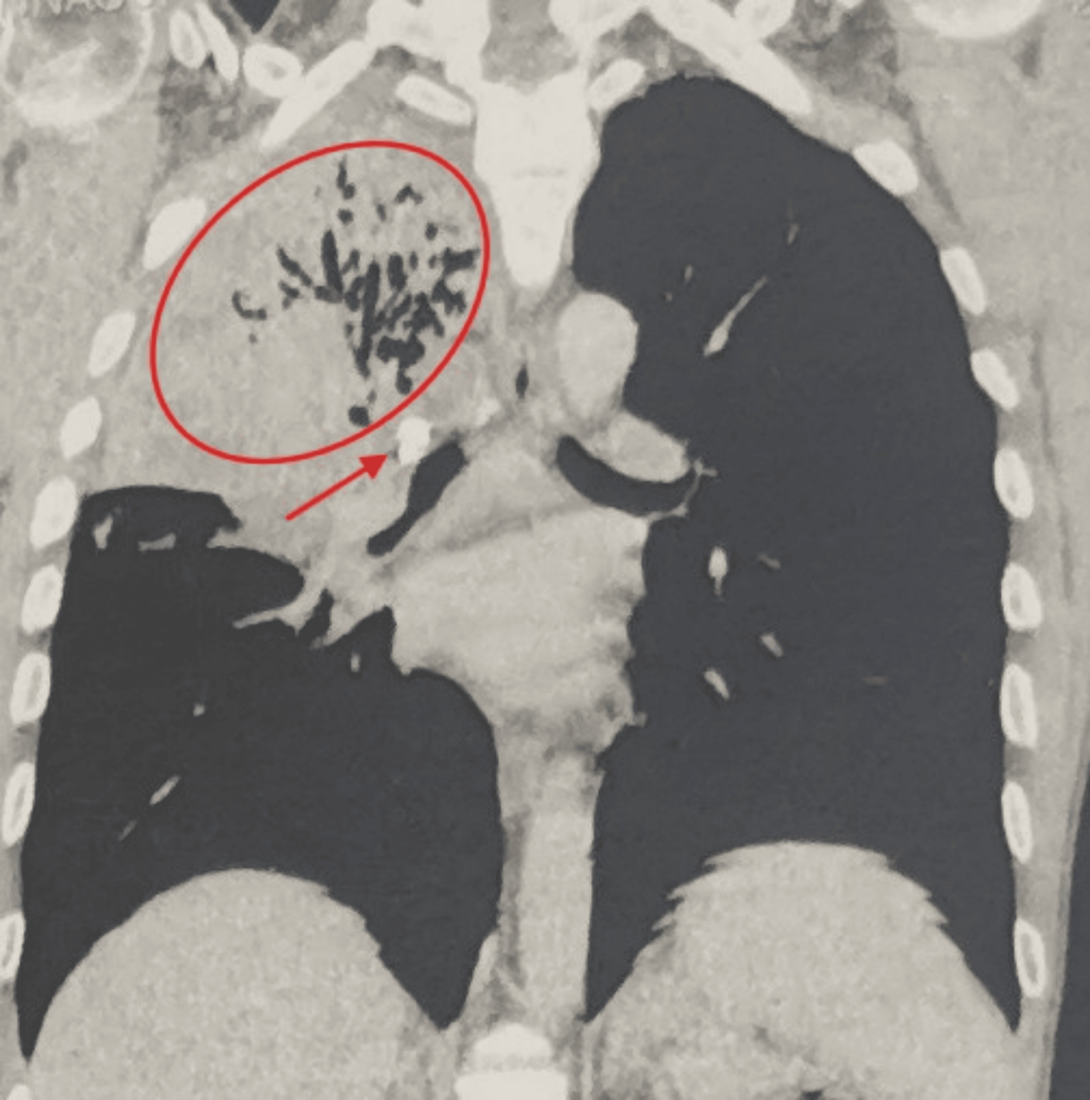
Coronal CT scan showing right upper lobe alveolar consolidation (red circle) with a calcified opacity in the right upper lobar bronchus (red arrow). CT, computed tomography
